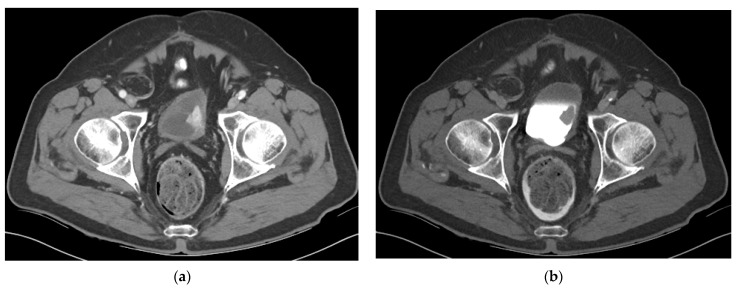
CT of a bladder mass during the urothelial phase and excretory phase (a) Axial CT image obtained during the urothelial phase shows a hyperenhancing bladder mass. Bladder tumors tend to be hypervascular and scanning the pelvis during the urothelial phase on CT may aid in tumor evaluation. (b) Axial CT image obtained during the excretory phase shows the same mass as a filling defect surrounded by excreted urine in the bladder.
